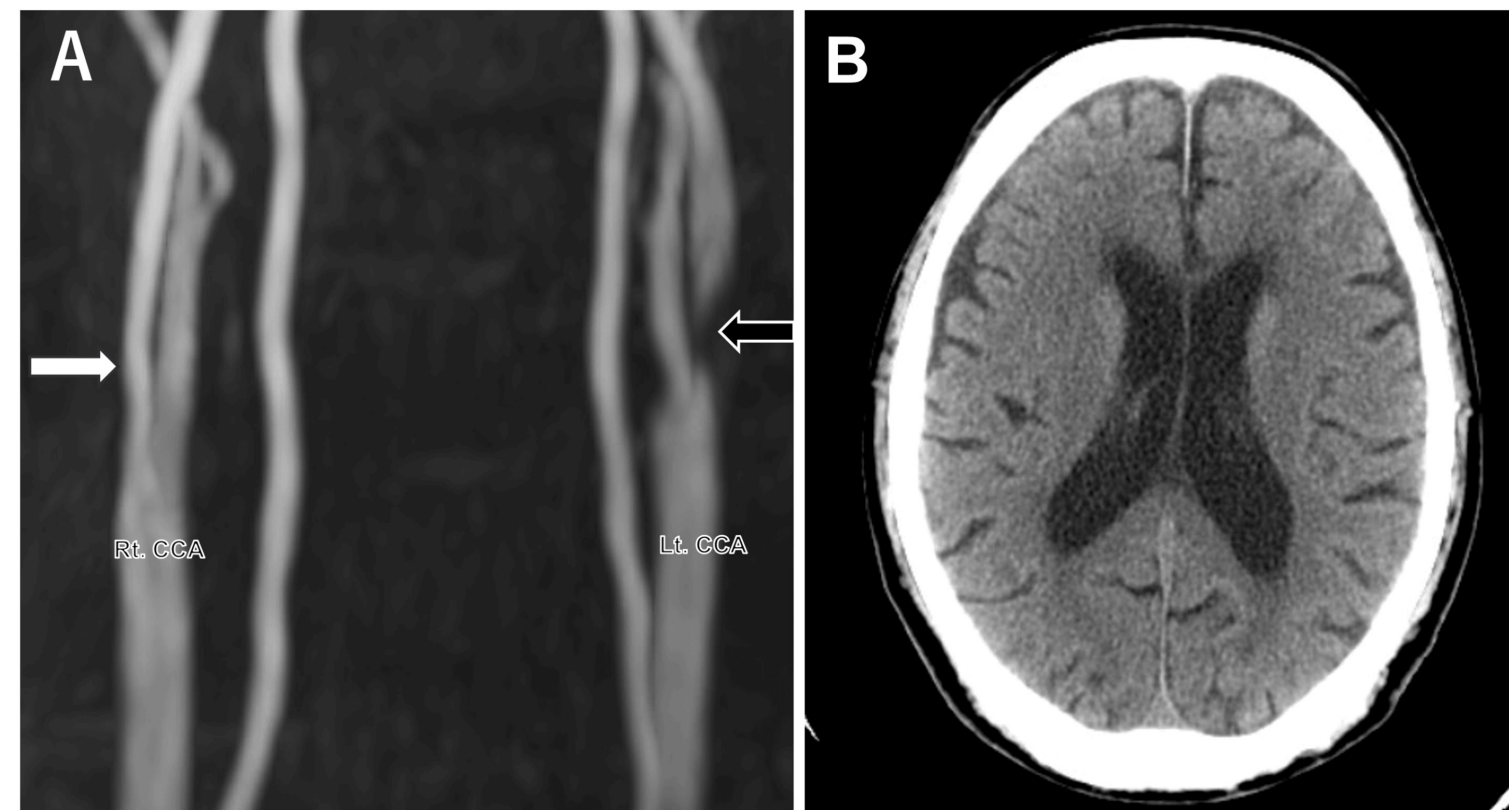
Radiological images at the initial intervention (A) Cervical MRA shows severe stenosis in the left ICA (black arrow), which was subsequently treated with carotid artery stenting. No stenosis is then identified in the right ICA (white arrow). (B) Non-contrast brain CT scan at that time identifies no evidence of low-density areas or cerebral calcifications. ICA: internal carotid artery, CCA: common carotid artery; MRA: magnetic resonance angiography; CT: computed tomography
